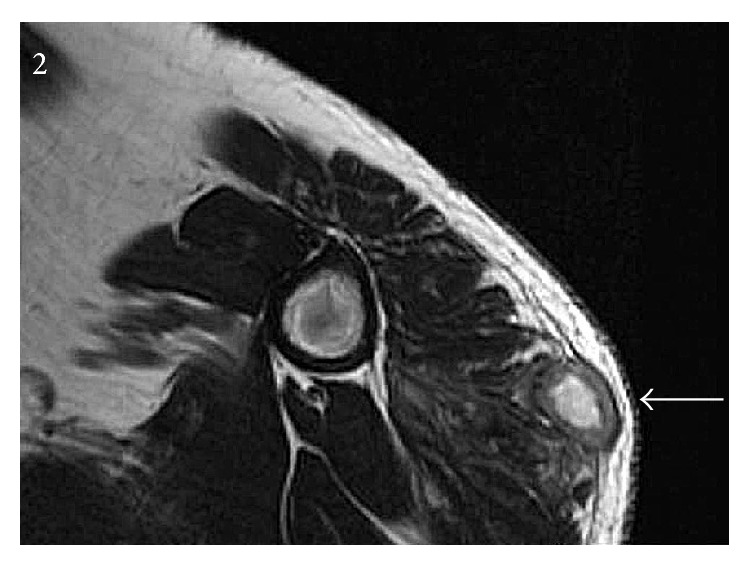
Magnetic resonance imaging (MRI) shows a complex, infiltrative maximally 9 cm mass in the left deltoid, extending from subcutaneous to deep tissues to abut the humerus, with underlying normal marrow. The lesion is composed of interlinked foci of abnormal tissue with intense central enhancement, suggesting necrosis.
